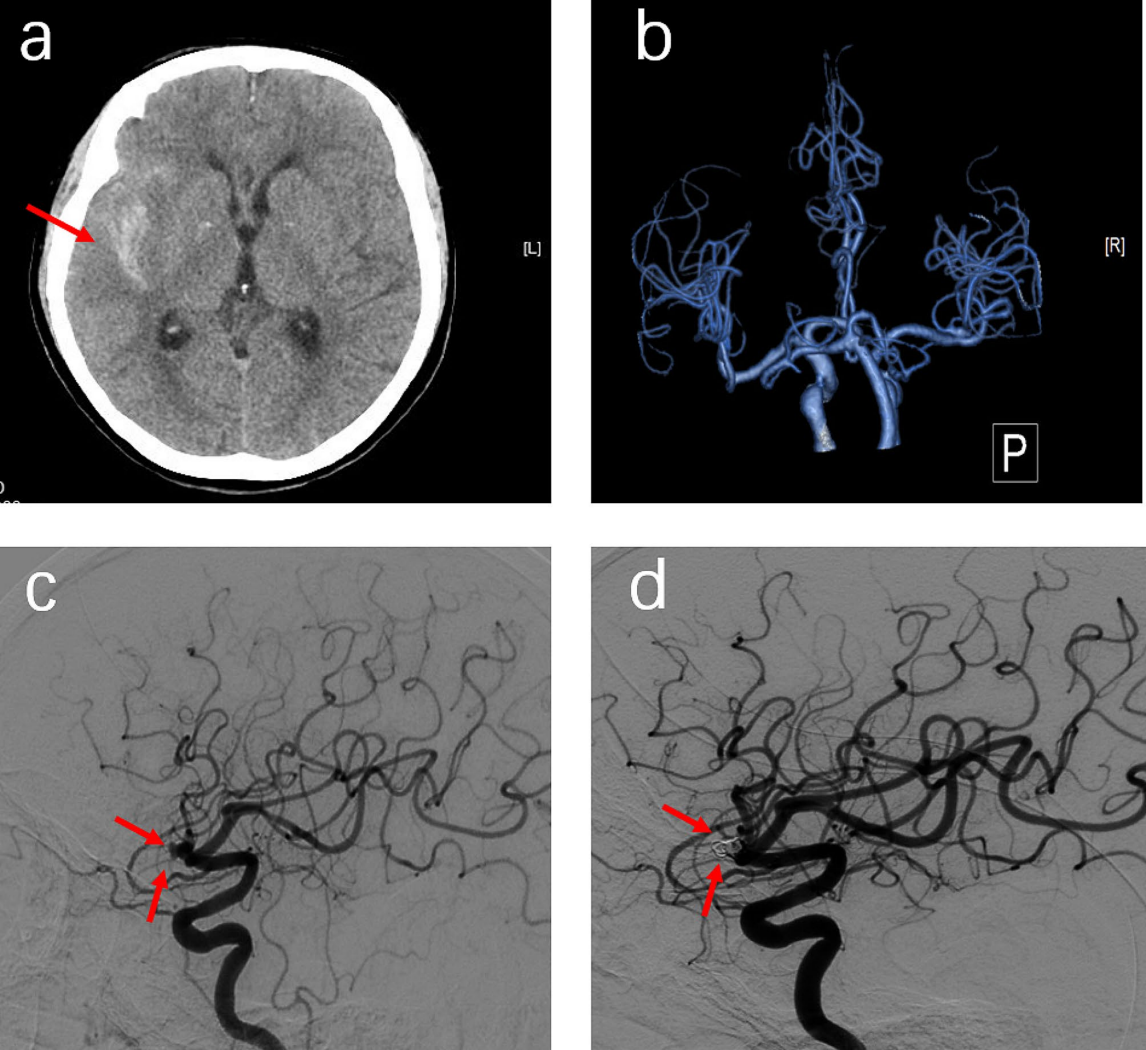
The imaging examinations before CIE. (a) Brain CT on the first day depicted a subarachnoid hemorrhage. (b) CTA detected no evident aneurysm or vascular malformation. (c) DSA revealed an aneurysm of 4 mm ∗ 3 mm in size in the M1 segment of the right MCA. (d) DSA demonstrated a coiling aneurysm embolization
